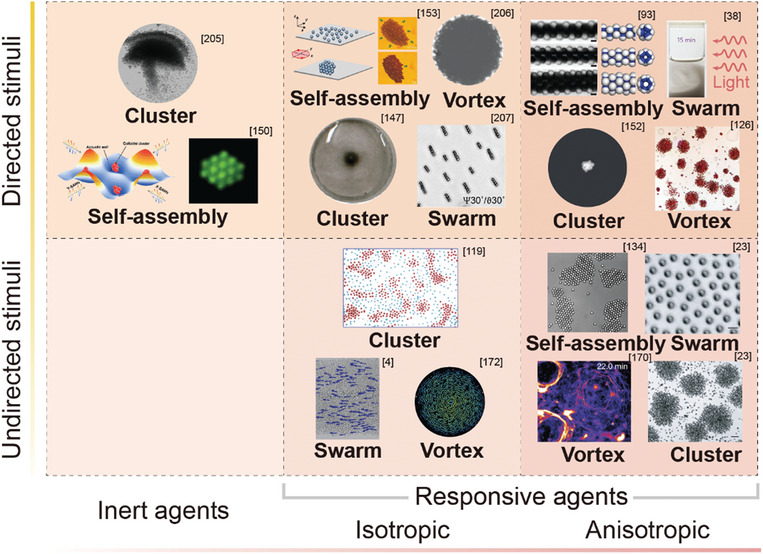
Summary of emergent swarm behaviors in synthetic swarms of active agents with distinct properties under different external stimuli. Cluster formation induced by ultrasound. Reproduced with permission.^[^
